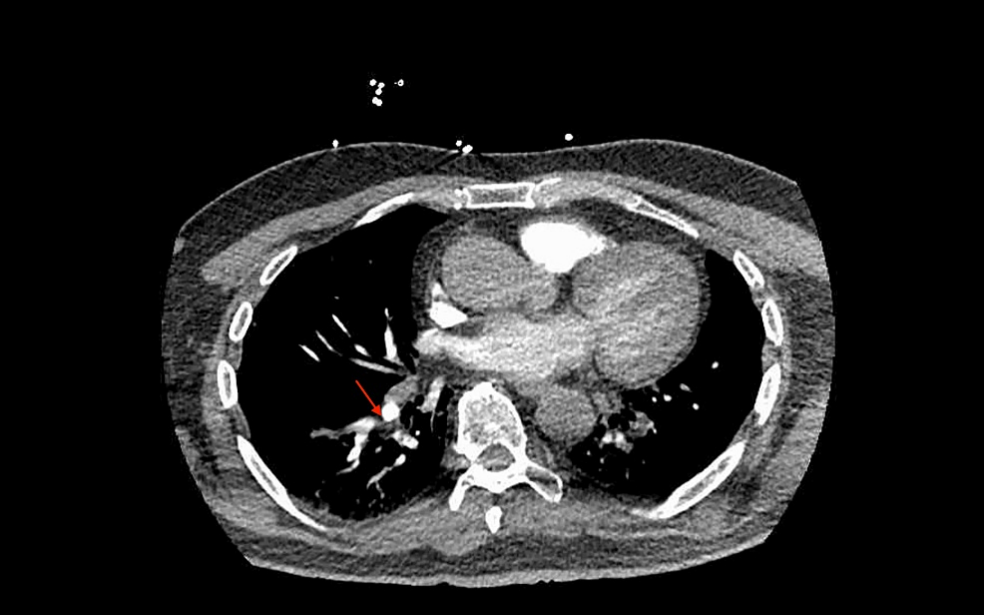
Computed tomography pulmonary angiography (CTA PE) showing right-sided segmental pulmonary embolus indicated with red arrow.
